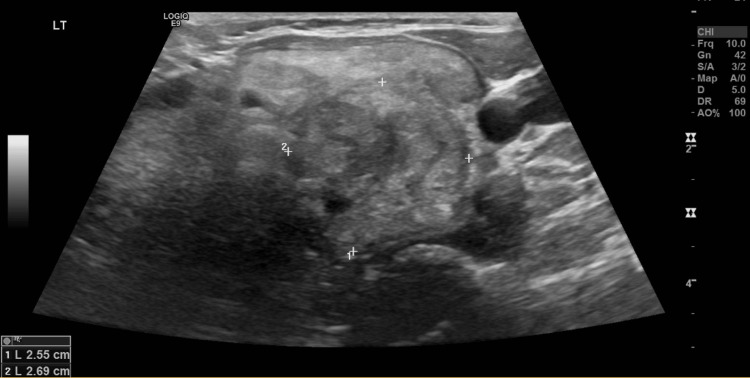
Left thyroid lobe ultrasonography showing a suspicious nodule
